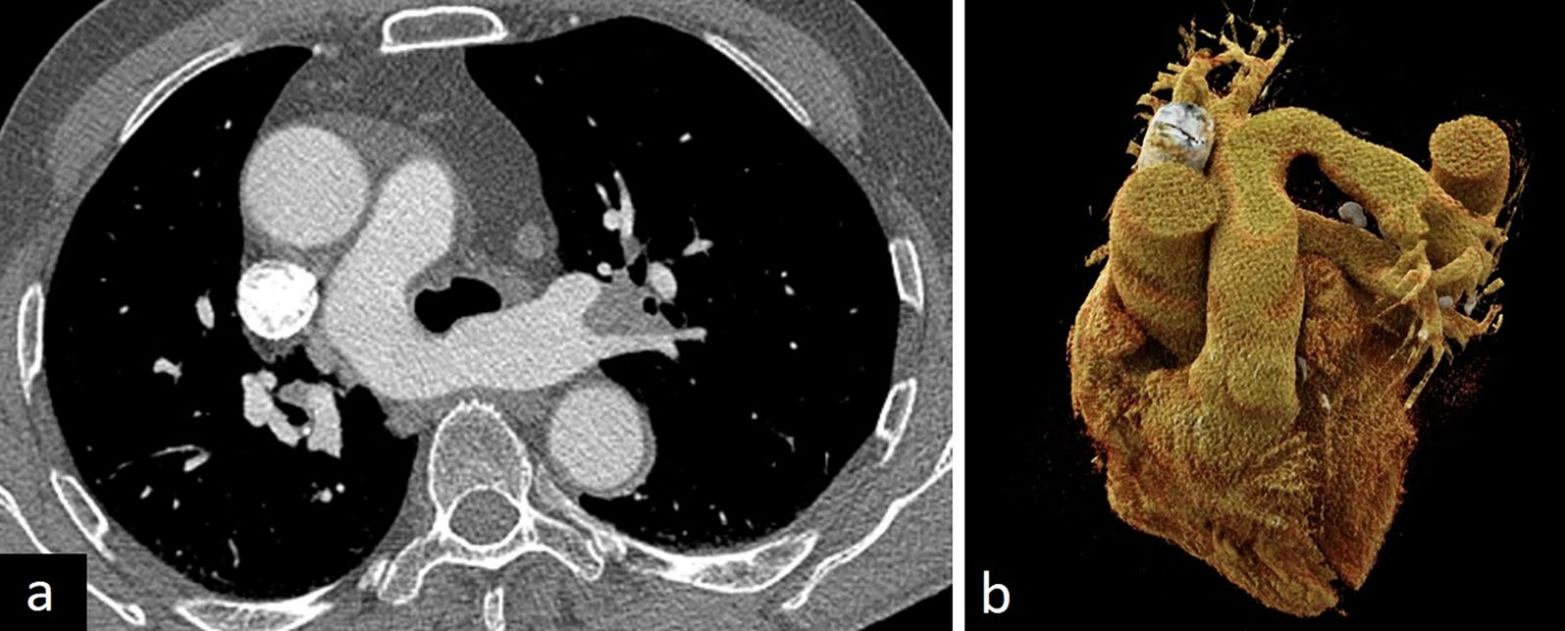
A 71-year-old asymptomatic male with pulmonary artery sling. Contrast-enhanced axial CT image (a) and 3D cinematic volume-rendered image (b) depict the aberrant left pulmonary artery arising from the right pulmonary artery and crossing to the left hemithorax
